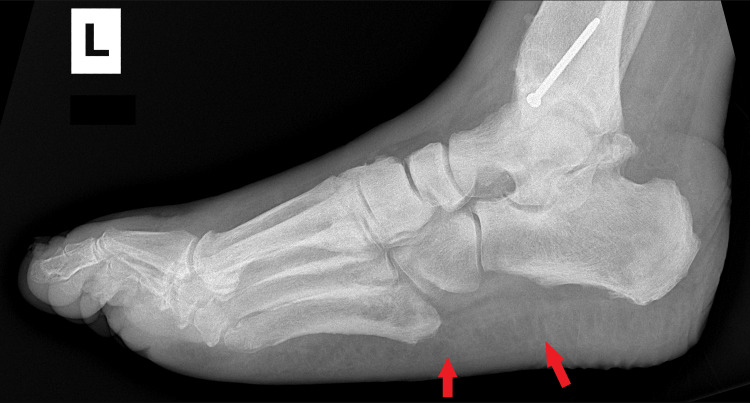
Lateral radiograph of the left foot demonstrating soft tissue edema in the region corresponding to the area of green discoloration noted on physical examination (red arrows). An orthopedic screw is visualized in the medial malleolus.
